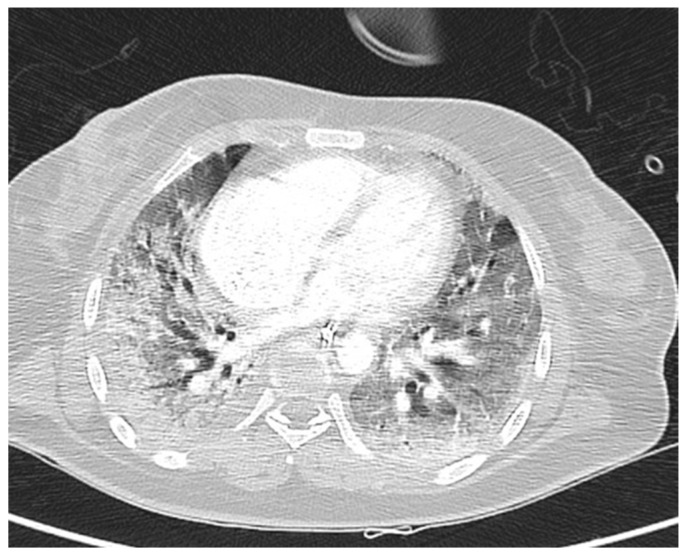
Bleomycin-related pulmonary toxicity: in this CT scan, bilateral severe fibrosis extended to all lung fields is represented. A 55-year-old woman experienced this fatal toxicity at the end of ABVD therapy for 6 cycles (with which she obtained CR).
